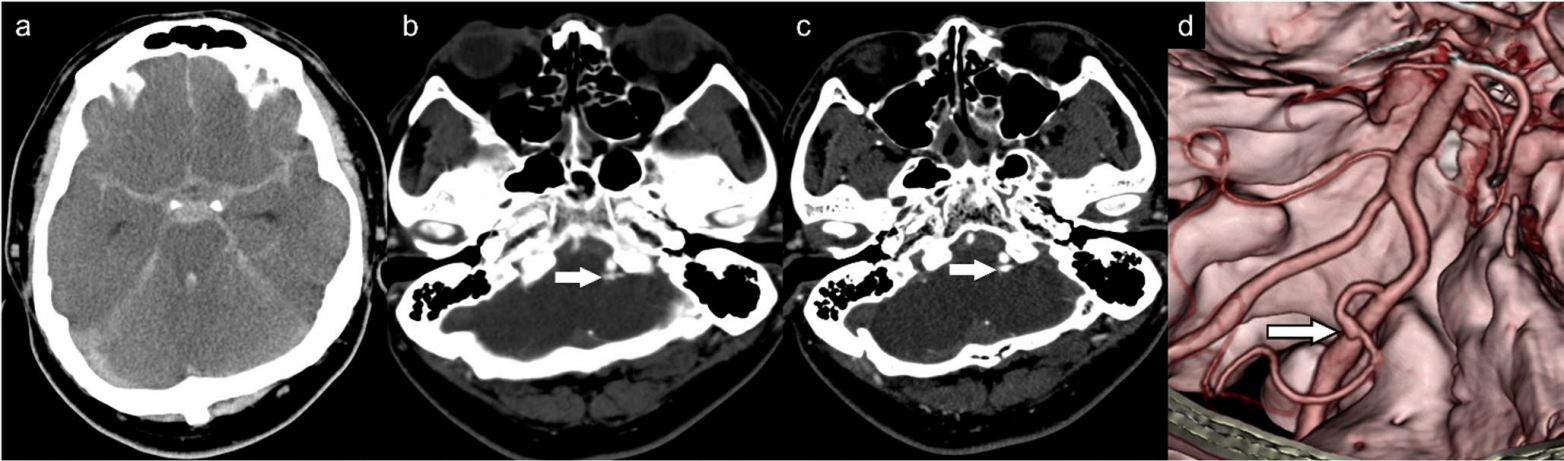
Left PICA pseudoaneurysm (a) NCCT shows massive SAH at the basal cistern with IVH. (b) 5-mm CTA fails to demonstrate a pseudoaneurysm and is misinterpreted as a non-specific vascular branch, as it was only visible in a single cut. (c) 0.5-mm CTA shows a focal dilatation at the proximal segment of the left PICA, visible on both axial and VRT images (d)
